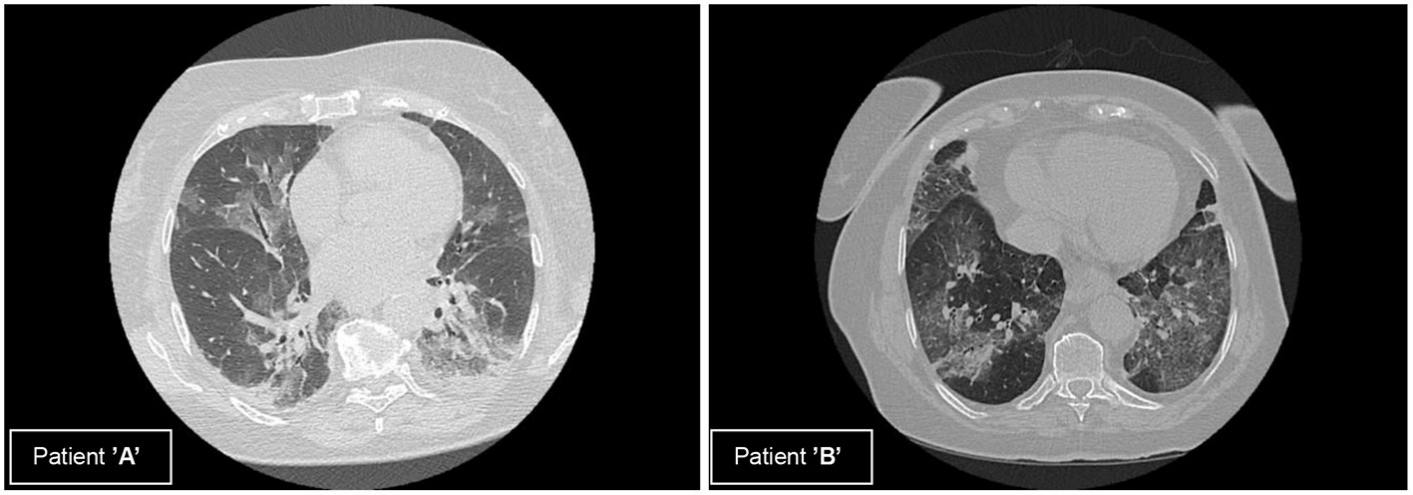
Computed tomography (CT) scans of patients (A) and (B). (A) Multifocal, subpleural, bilateral ground glass opacities with subpleural traction in the dorsal regions. (B) Multifocal, subpleural, bilateral ground glass opacities, crazy paving dominantly on the left side, consolidation in the right basal region.
